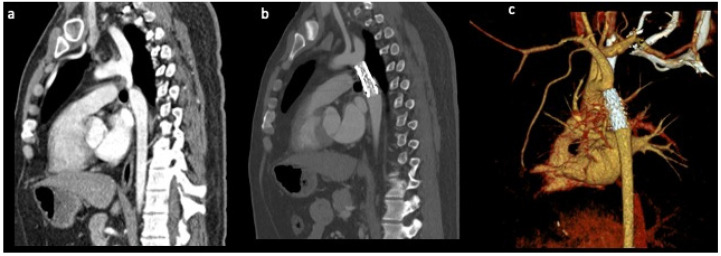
Computed tomography angiography of the aorta in the sagittal planes demonstrating (a) the severity of the coarctation (b) and after successful stenting. (c) Three-dimensional reconstruction displaying the luminal surface of the Cheatham-Platinum covered stent.
